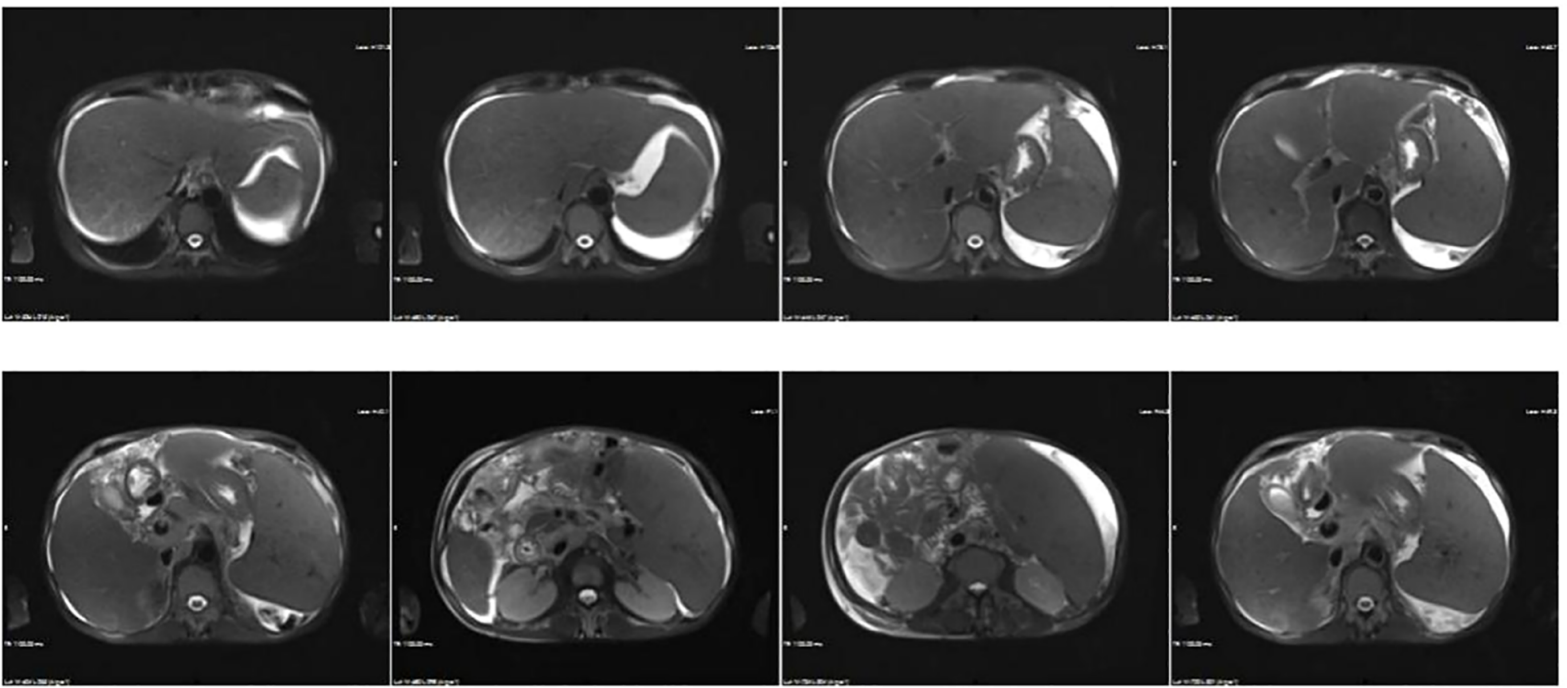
Abdominal MRI displaying heterogeneous liver signals, suggestive of possible cirrhosis and portal hypertension. Additional findings include cholecystitis with cholestasis, splenomegaly, ascites, and intestinal tube abnormalities.
